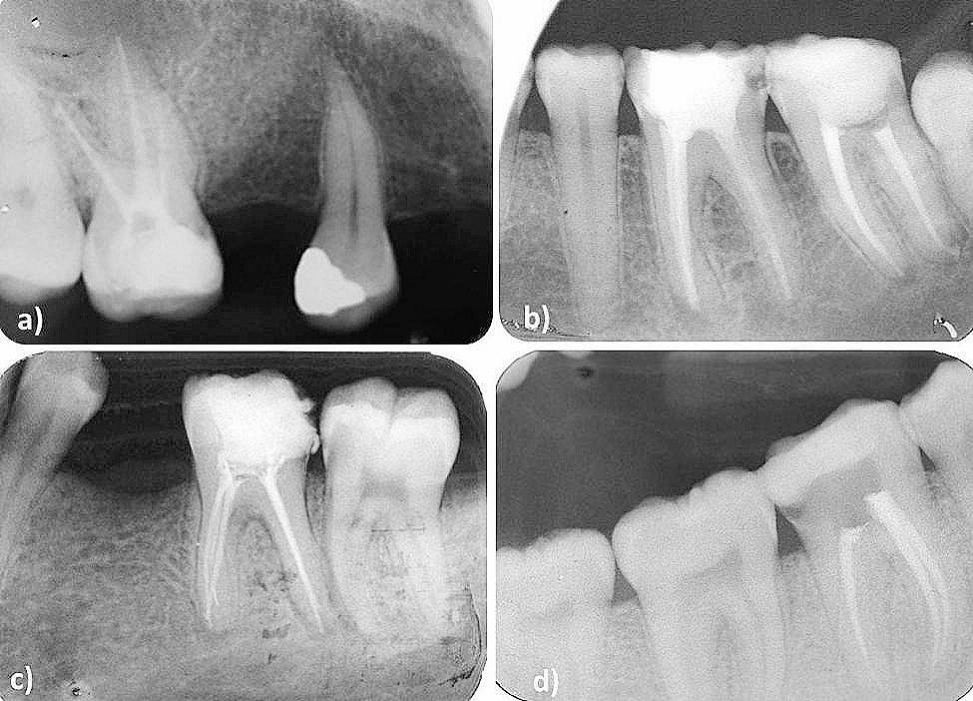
Procedural errors detected in the post-obturation radiographs; (a) under-extension of obturation in the mesio-buccal root of the maxillary right first molar, (b) canal transportation in the mesial root of the mandibular left first molar, (c) separated instrument in the mesio-lingual root of the mandibular left first molar, and (d) Defective coronal restoration in the mandibular right first molar
